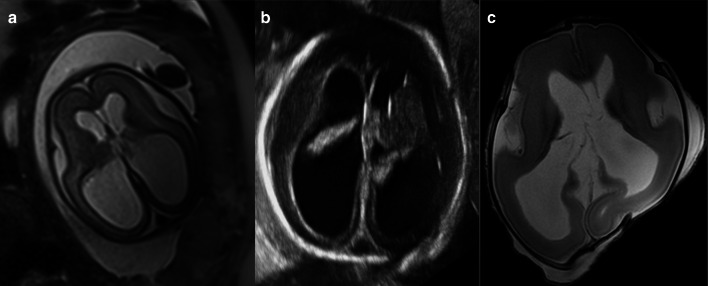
26-week, elective termination of pregnancy due to ventriculomegaly. a Intrauterine HASTE MRI sequence showing the ventricular dilation. b Intrauterine ultrasound shows the same findings and (c) axial post-mortem T2W MRI demonstrating the ventricular dilation
